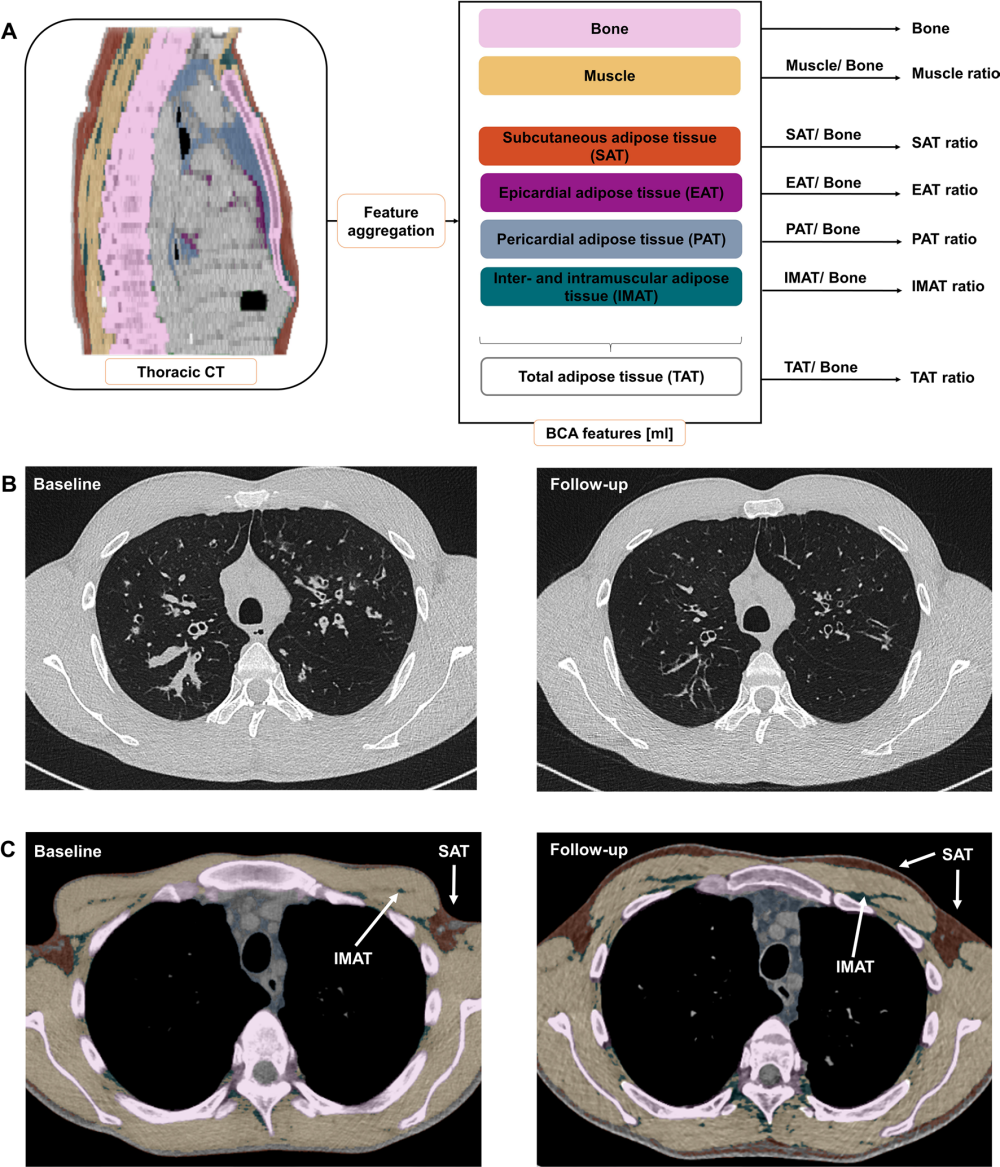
Exemplary in- and output of fully automated CT-based body composition analysis (BCA). (A) Visualization of feature extraction for BCA and marker aggregation. BCA network detects the different BCA features within the chest CT scan. Those raw features are combined with bone to calculate body size-adjusted biomarkers. The tissues are encoded in colors as follows: pink: bone, yellow: muscle, orange-brown: subcutaneous adipose tissue (SAT), purple: epicardial adipose tissue (EAT), light blue: paracardial adipose tissue (PAT) and turquoise: inter- and intramuscular adipose tissue (IMAT). (B) Exemplary chest CT in axial view before (left) and after (right) elexacaftor/tezacaftor/ivacaftor therapy showing decreasing bronchiectasis wall thickening and regredient mucus impaction. (C) Exemplary chest CT in axial view showing increased proportion of subcutaneous adipose tissue (SAT, orange-brown) and increased inter- and intramuscular adipose tissue (IMAT, turquoise) after elexacaftor/tezacaftor/ivacaftor therapy.
